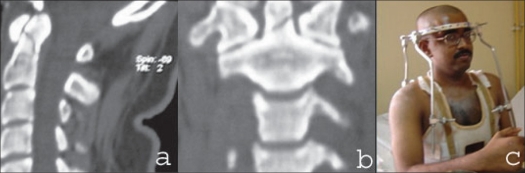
Reconstructed sagittal CT scan (a) and coronal CT scan (b) shows undisplaced type 2 fracture of the odontoid in a 26 years old male. The patients was treated (c) conservatively with halo vest immobilization
